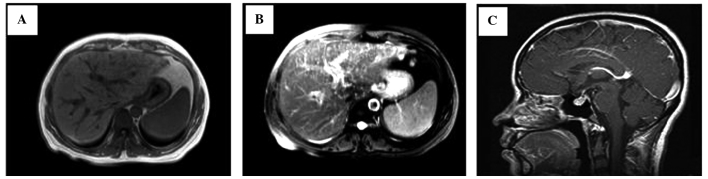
Contrast-enhanced magnetic resonance imaging (MRI) (A and B) T1 signal of the liver. (A) Prior to the injection of the contrast agent and (B) following the injection of a contrast agent. (C) T2 signal of the head.
